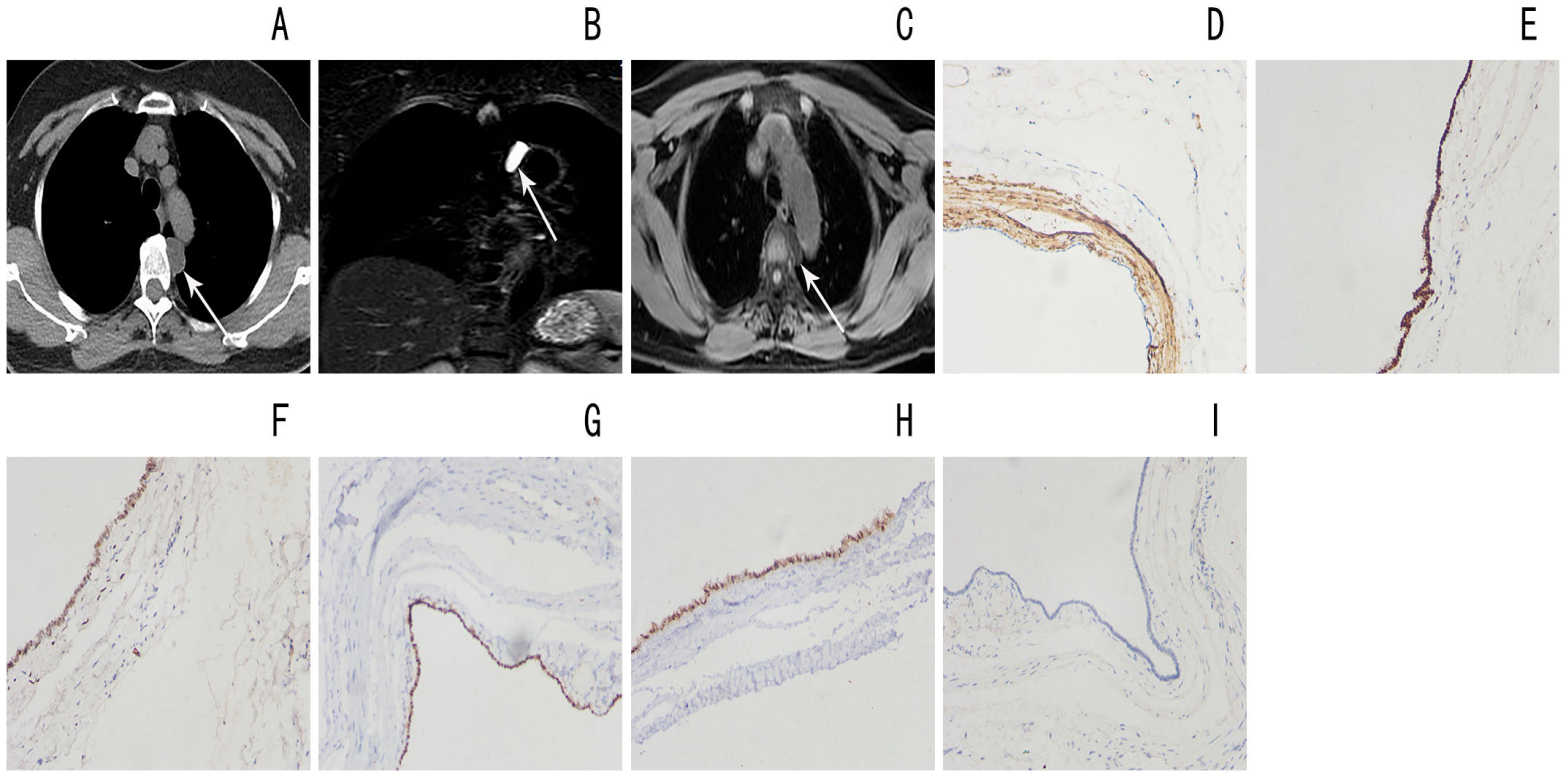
(A) Chest CT shows a low-density mass in the left posterior mediastinum with clear boundary (arrow) (B, C) Magnetic resonance imaging shows a long T1 long T2 cystic lesion in the left posterior mediastinum with well-defined borders (arrow) (D) Diffuse SAM positivity in the cyst wall lining epithelium High magnification (E) The epithelium lining the cyst wall is diffusely positive for estrogen receptor (ER) High magnification (F) The epithelium lining the cyst wall is diffusely positive for progesterone receptor (PR) High magnification (G) Diffuse WT1 positivity in the cyst wall lining epithelium High magnification (H) Diffuse PAX8 positivity in the epithelium lining the cyst wall High magnification (I) Low value-added Ki-67 in the cyst wall lining epithelium High magnification.
